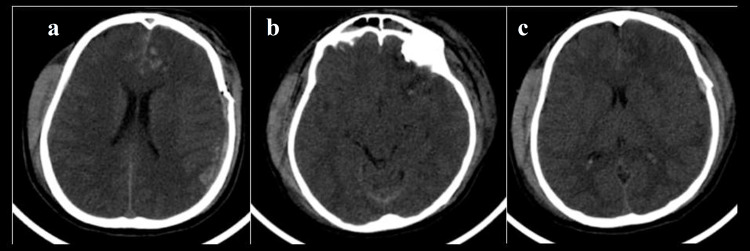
CT of a 22-year-old male patient following road traffic accident Initial admission CT shows (a) soft tissue swelling in the right parietal region with extradural hemorrhage in the left parietal convexity and traumatic subarachnoid hemorrhage in bilateral frontal lobes - Rotterdam score 3. Sequential CTs after 24 hours show (b) effacement of basal cisterns and (c) cerebral sulcal spaces - Rotterdam score 4.
